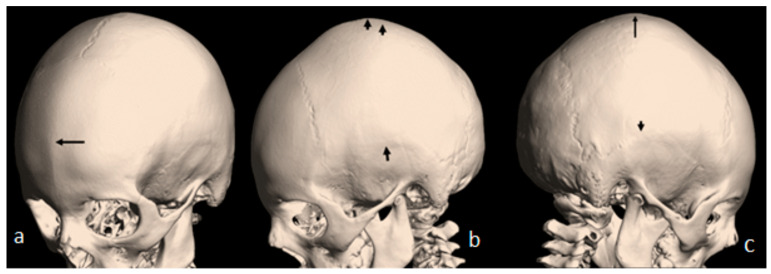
(a) A 3D reconstruction CT scan of the 7-year-old-child with mild flexion of the skull showed early closure of the metopic suture (arrow). (b) A 3D reconstruction CT scan showed early closure of the squamosal sutures (arrow), pressing the brain contents upward, causing the development of a prominent bulge at the top of the mid-sagittal sutures two arrows. (c) Another 3D reconstruction CT scan confirmed the bilateral closure of the squamosal suture (arrow head). The vertical arrow showed abnormal bulging of the vertex secondary to bilateral pressure exerted by the squamosal sutures.
